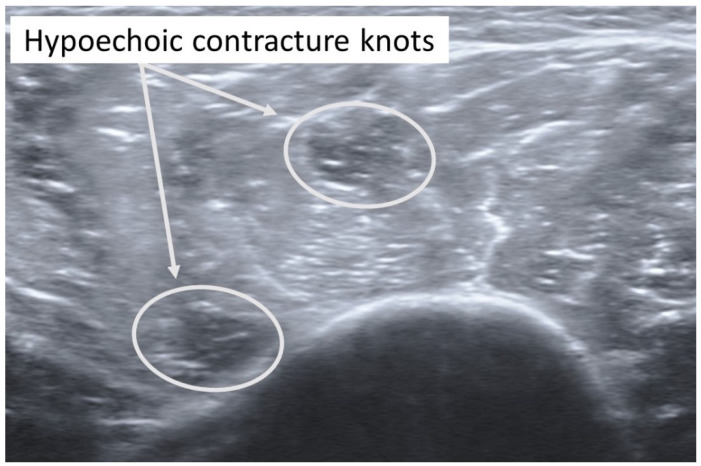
Ultrasound imaging assessment of the right deltoid muscle showing two palpable contracture knots as hypoechoic (hyperperfused) areas.
